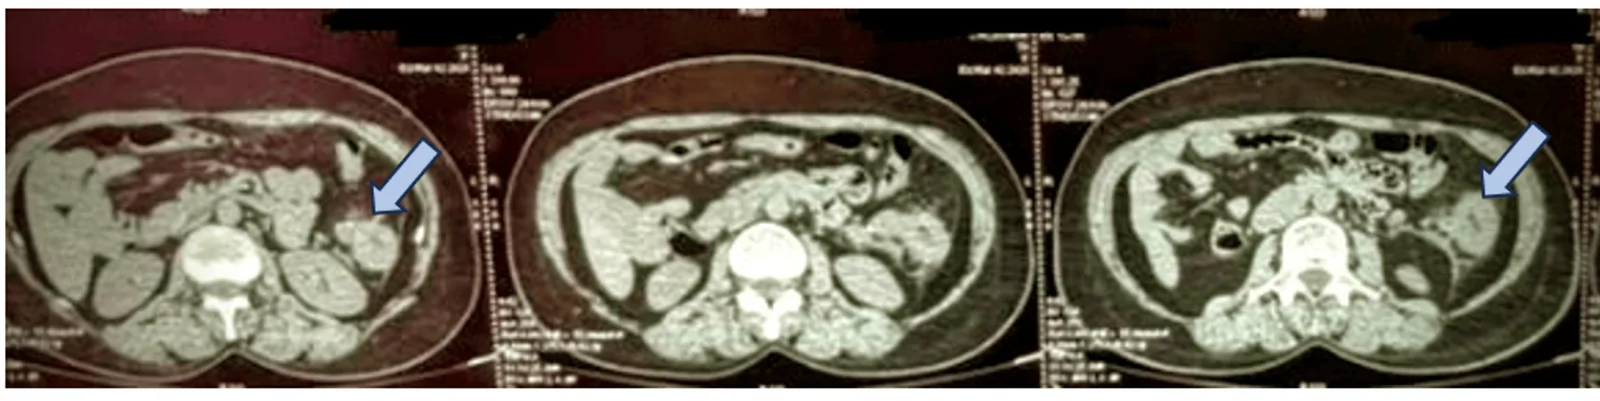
CECT showing colonic wall thickening and fat stranding The figure shows a CECT of the abdomen scan taken prior to surgery, which showed circumferential enhancing wall thickening and fat stranding of the descending colon (indicated by the marked arrows), suggestive of a colonic carcinoma. CECT: contrast-enhanced computed tomography
